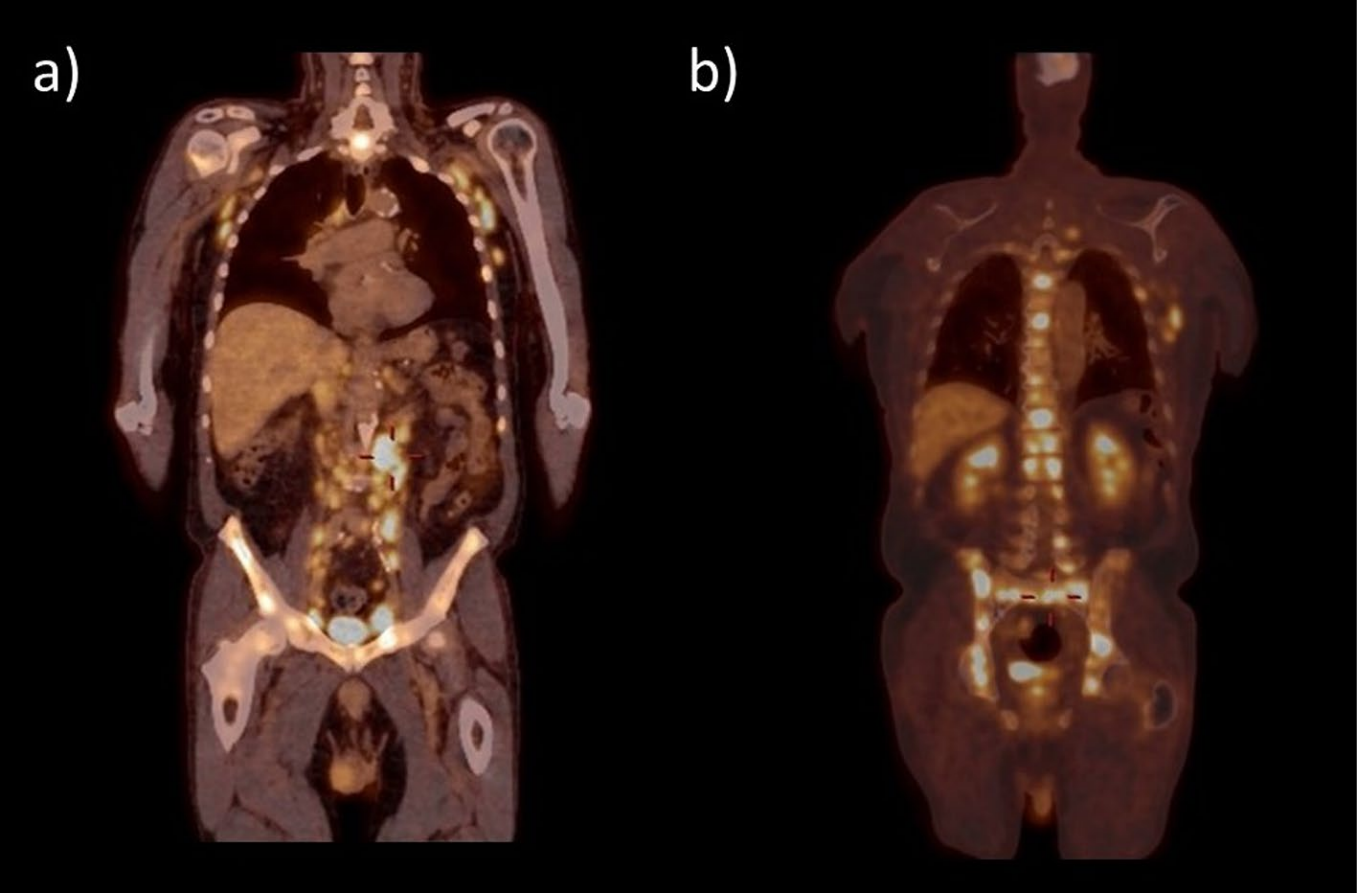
Whole body ^18^F-FDG PET/CT demonstrating hypermetabolic lesions diffusely in the lymph nodes (a) and bones (b), highly suggestive of a high-grade relapse of the lymphomatous disease
